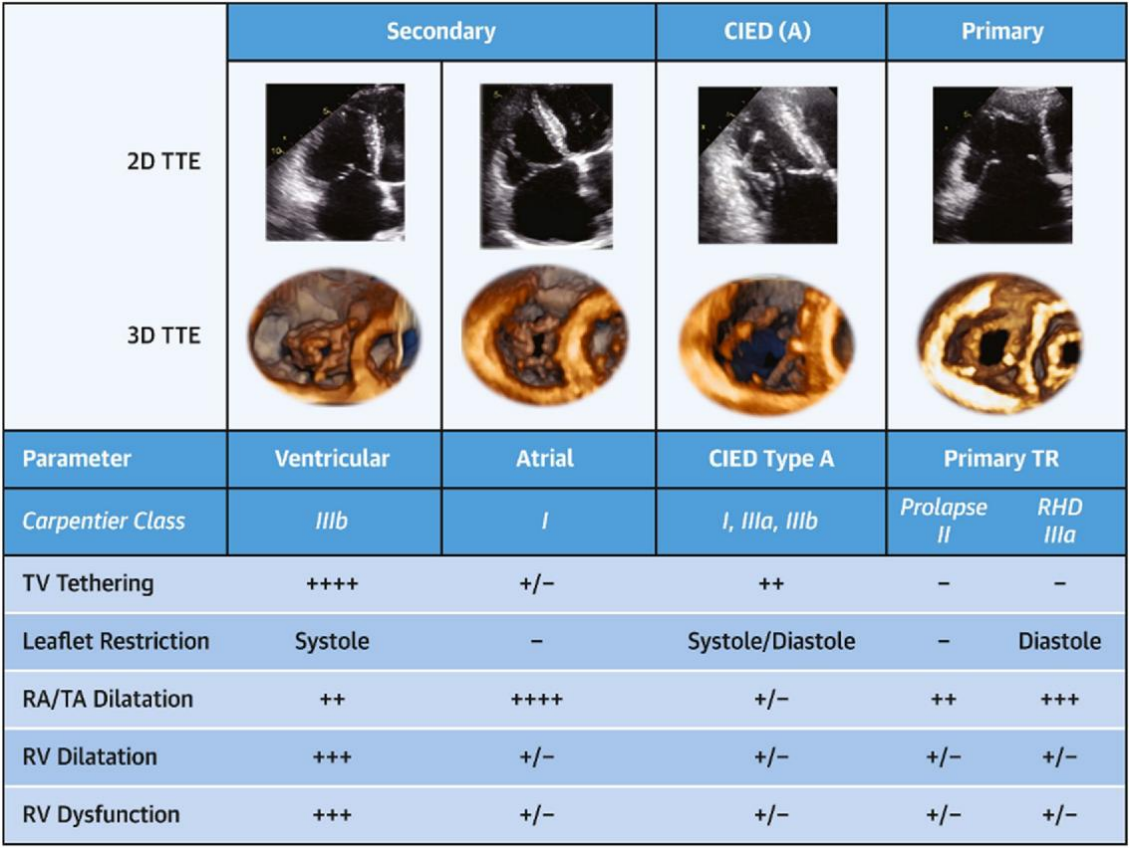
Classification of TR aetiology (reprinted from^18^, with permission from Elsevier). The expanded tricuspid regurgitation (TR) classification by aetiology currently separates secondary TR into atrial secondary and ventricular secondary disease. When cardiac implantable electronic device (CIED) leads are present, lead-associated TR may be sub-categorized into type A, whose CIED is causing the TR, and type B, whose CIED is incidental. 2D, 2-dimensional; 3D, 3-dimensional; RA, right atrium; RV, right ventricle; TA, tricuspid annulus; TTE, transthoracic echocardiography; RHD, rheumatic heart disease; TV, tricuspid valve.
